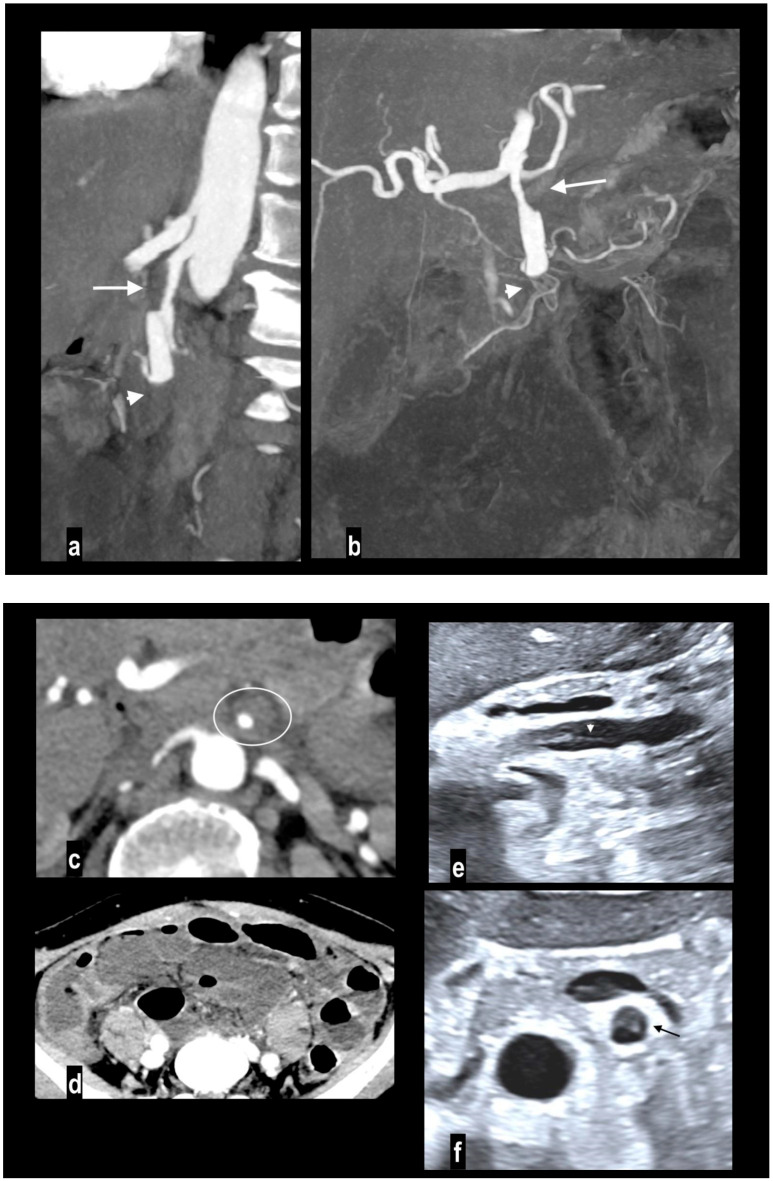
SMA dissection in a 42-year-old woman. MIP arterial images (a,b) showed segmentary false lumen thrombosis in the proximal convex side of SMA, with lumen narrowing (arrow). The vessel caliber had increased ((c) oval). Distally, the intimal flap continued in a complete vessel thrombosis (arrowhead), determining bowel ischemia with hypoenhancing paper-thin bowel walls (d). Proximal false lumen thrombosis (arrow) and intimal flap (arrowhead) were also well depicted at ultrasound examination performed before MDCT (e,f). The patient underwent surgical resection of necrotic loops.
